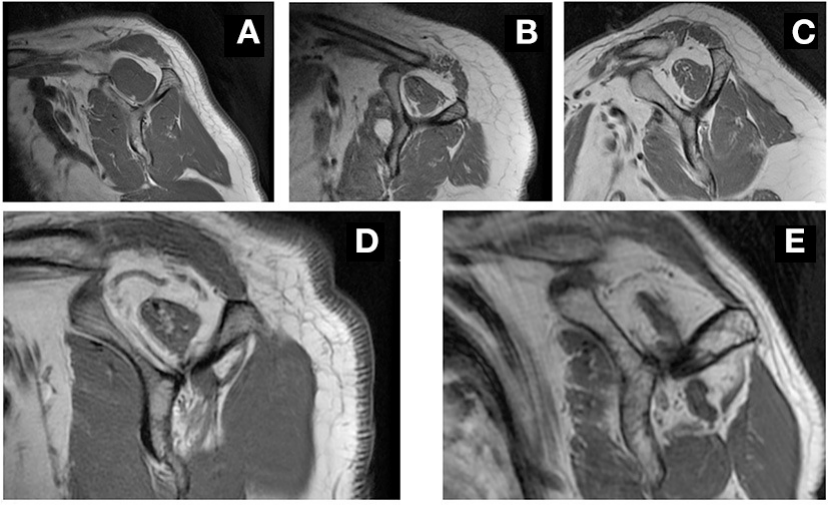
Representative MRI image in grading fatty infiltration. Sagittal oblique views in T1-weighted MRI, showing grade 0 (A), grade 1 (B), grade 2 (C), grade 3 (D) and grade 4 (E) fatty infiltration of the supraspinatus muscle.
